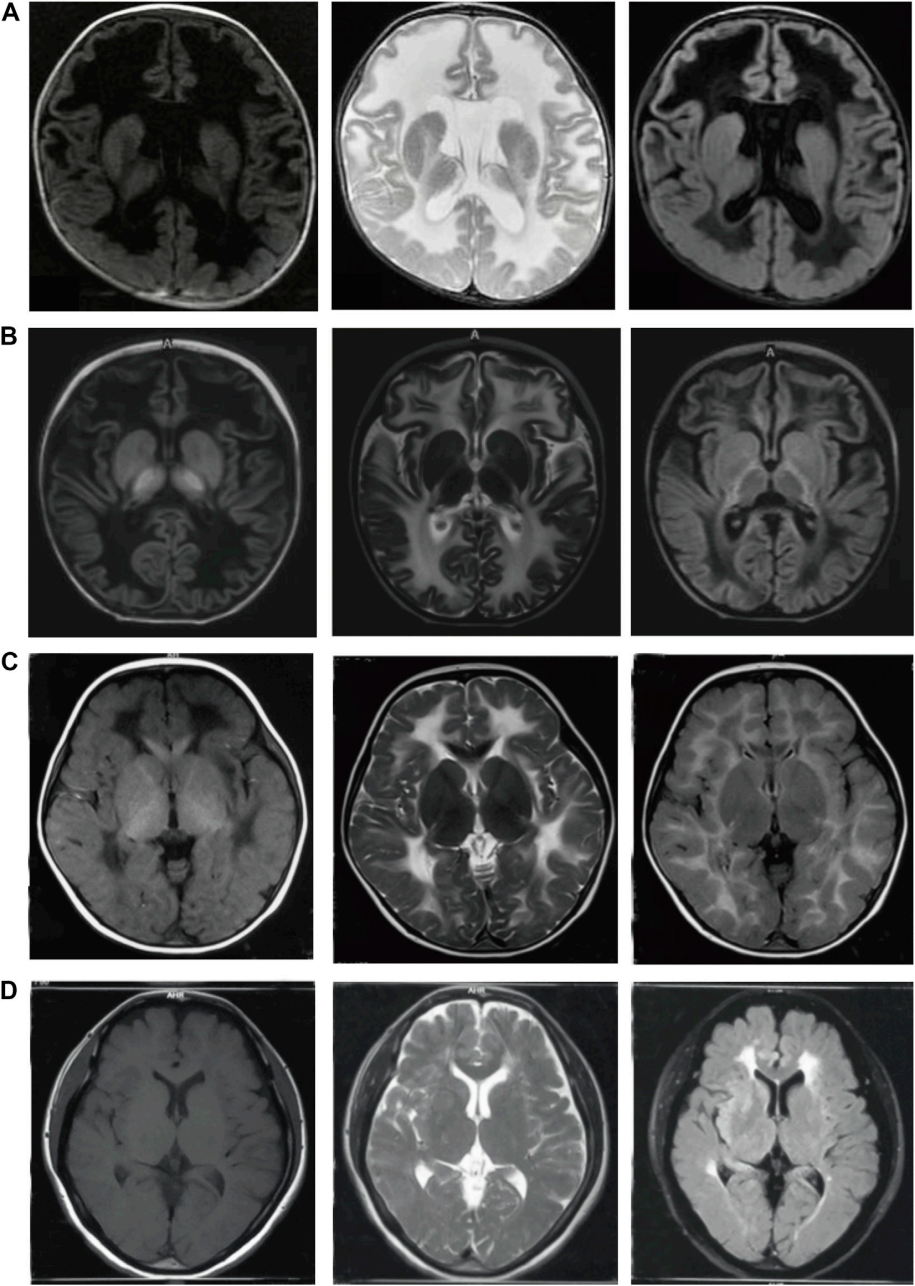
Brain MRI of four patients with VWM at different onset ages. (A): case 1 with onset in neonatal period, the age at MRI was 3 months; (B): case 2 with onset in infancy, the age at onset was 11 months, the age at MRI was 18 months; (C): case 3 with onset in childhood, the age of onset was 3 years old, the age at MRI was 3 years old; (D): case 4 with onset in adulthood, the age of onset was 43 years old, the age at MRI was 43 years old. The left column:T1 weighted sequences (T1WI); The middle column:T2 weighted sequences (T2WI); The right column: T2 FLAIR sequences. The white matter showed low signal on T1WI, high signal on T2WI and low signal liquefaction on T2 FLAIR. The earlier the age of onset, the wider the range of white matter liquefaction, while the liquefaction sign was not evident in adult patients. Furthermore, the subcortical white matter was involved in the early stage.
